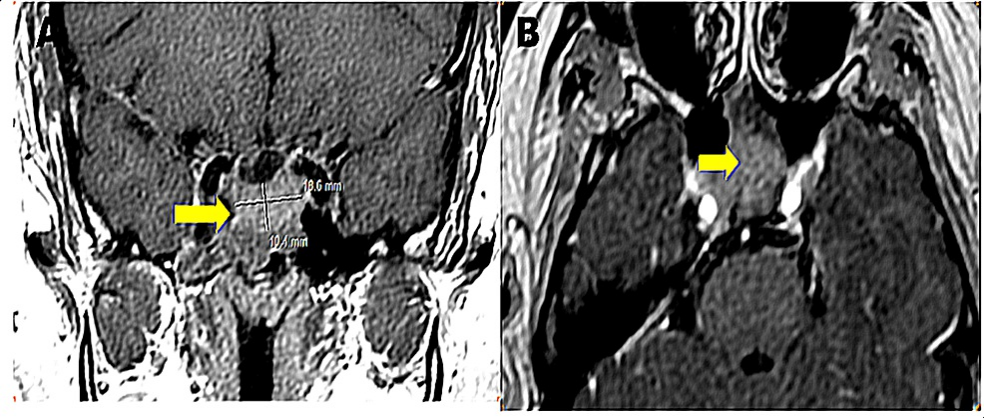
Injected MRI images: coronal T1 (A) and axial T2 (B) showing a pituitary macroadenoma invading the cavernous sinus and enveloping the right internal carotid artery. It also invades the sellar bottom and the large wing of the right sphenoid (Department of Radiology CHU Hassan II Fez).
